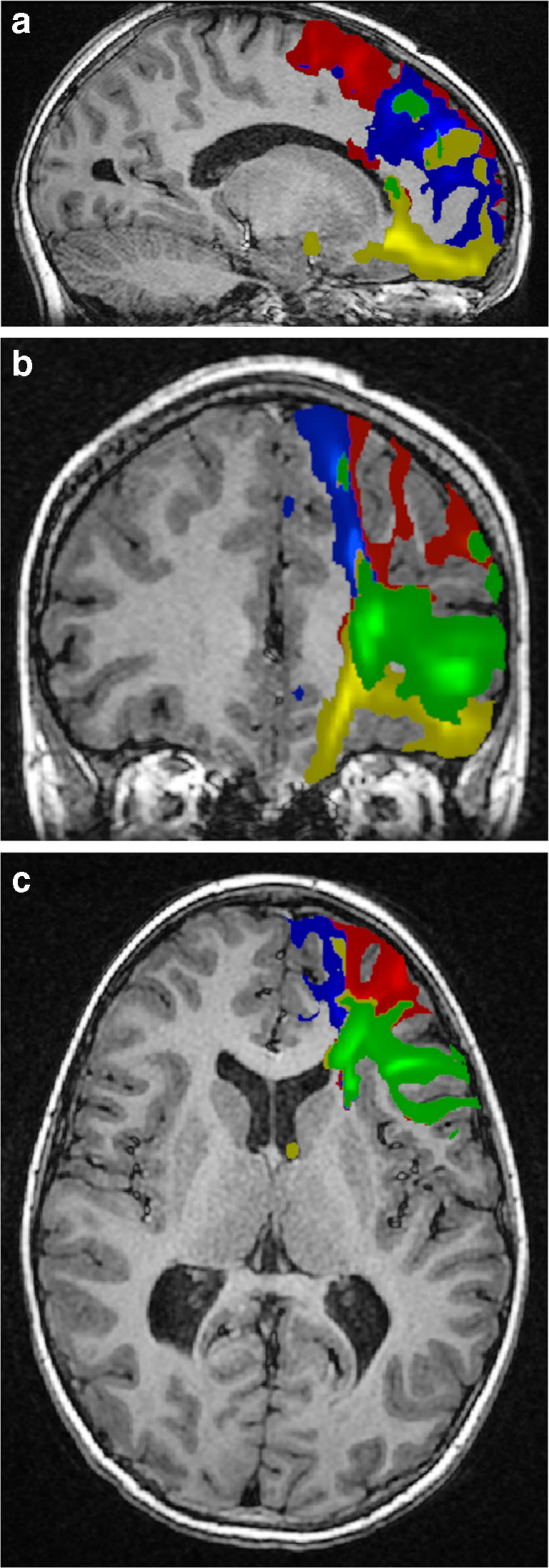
T1-weighted MR images with an overlay of the frontostriatal tracts coded by colours in a 9.1-year-old healthy boy (a control) who had normal findings at conventional MRI. a Sagittal. b Coronal. c Axial. The dorsolateral prefrontal caudate tract is red, the medial prefrontal caudate tract is blue, the orbitofrontal caudate tract is yellow and the ventrolateral prefrontal caudate tract is green
